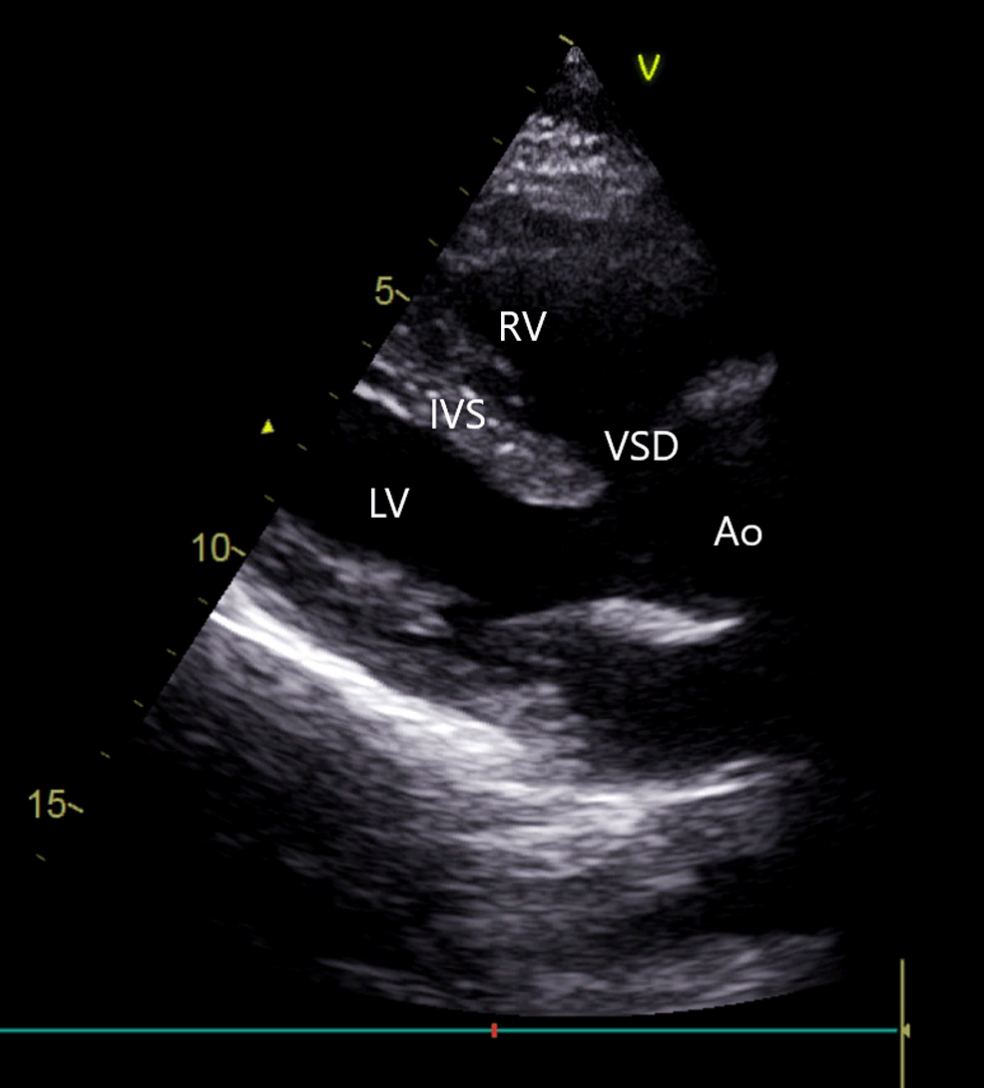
Transthoracic echocardiography showing large sub-aortic ventricular septal defect with overriding of the aorta with severe valvular pulmonary stenosis and right ventricular hypertrophy. RV = right ventricle, LV = left ventricle, IVS = interventricular septum, VSD = ventricular septal defect, Ao = aorta
